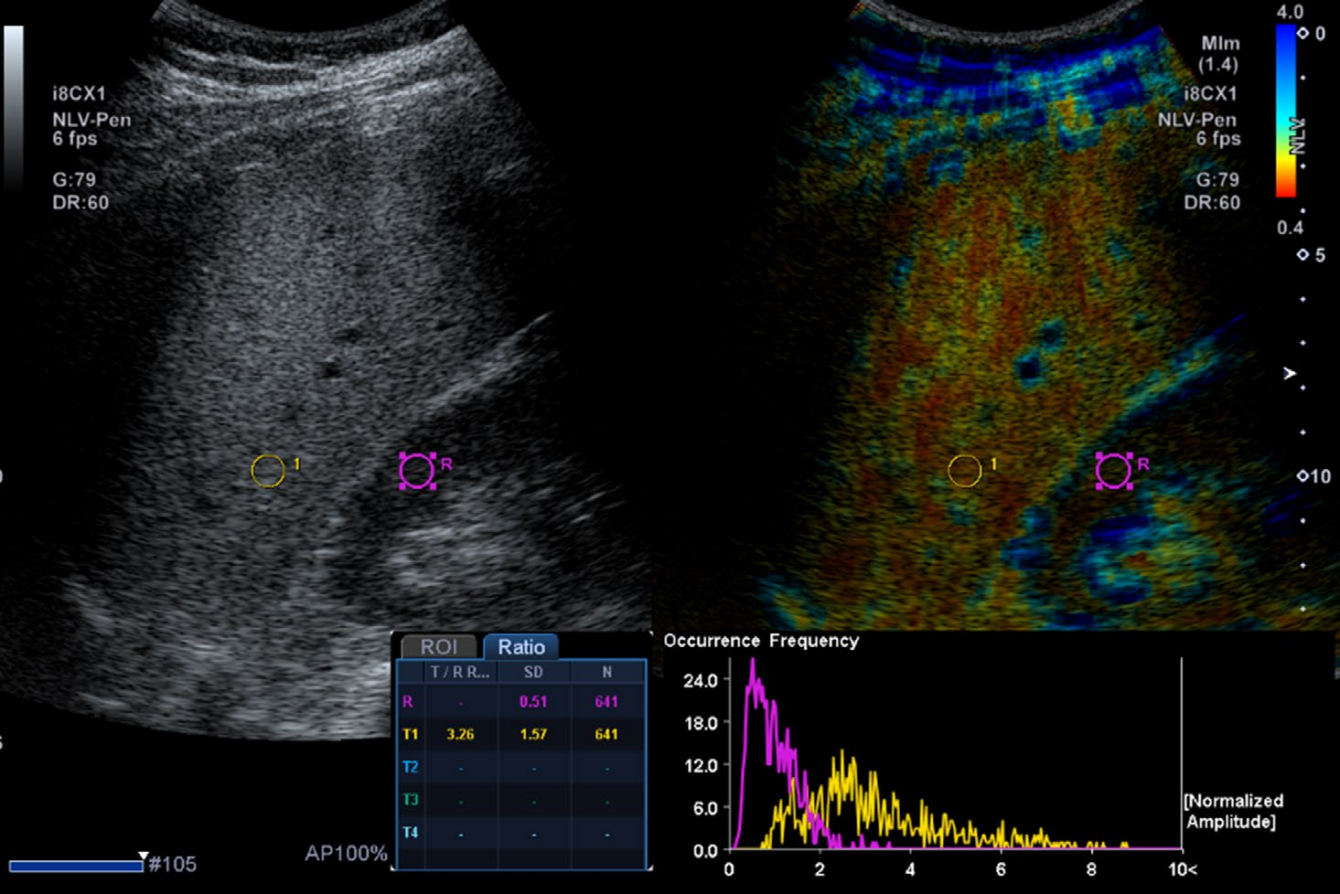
Longitudinal gray‐scale ultrasound image of a 13‐year‐old boy on the left shows echogenic, homogeneous appearance of the liver, consistent with hepatic steatosis. Left gray‐scale image and right color images show placement of small regions of interest in the liver and right kidney parenchyma for automated hepatorenal index calculation.
